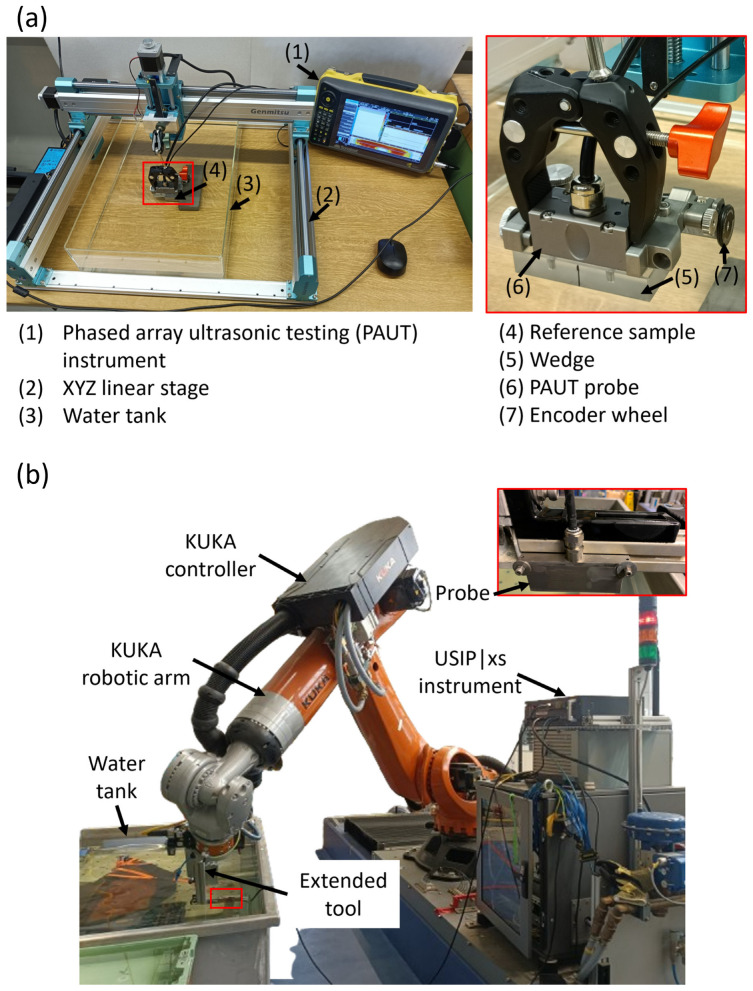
(a) Phased array testing setup (Sonatest VEO system) used for testing W/EUROFER FGM-coated flat sample, and (b) Krautkrämer HydraStar robotic ultrasonic system used for testing L-shape 50% W and 50% EUROFER curve coated sample. The inset red box shows the magnified view of the probe.
